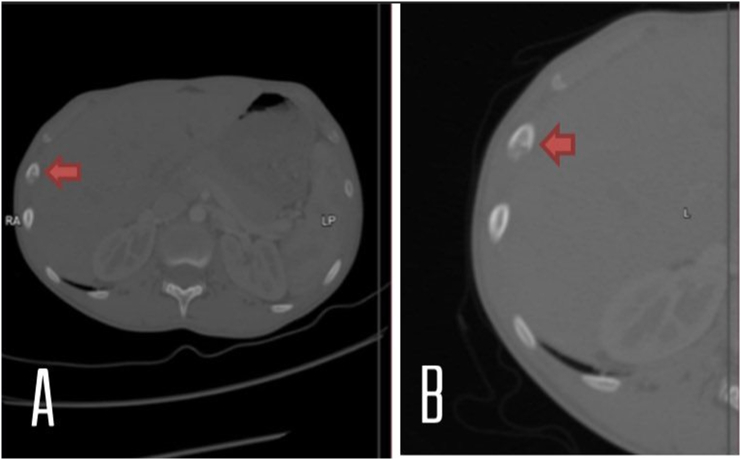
Preoperative computed tomography. Axial view (A) and zoomed in Axial view (B). A well-demarcated 5-mm osteolytic lesion with central calcification can be observed in the right 9th rib without any periosteal reaction.
